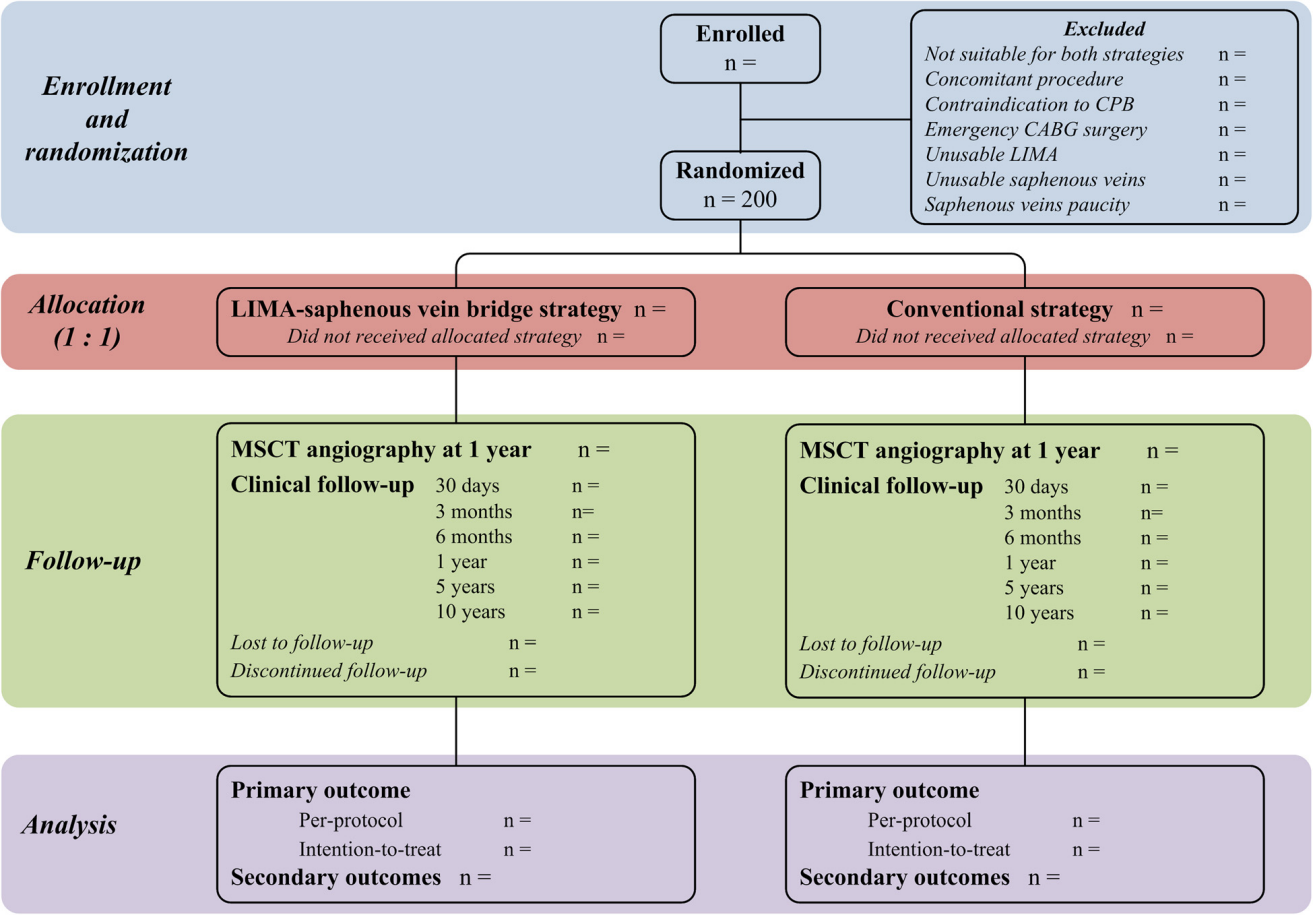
Study design. CABG, coronary artery bypass grafting; CPB, cardiopulmonary bypass; LIMA, left internal mammary artery; MSCT, multislice computed tomography.
